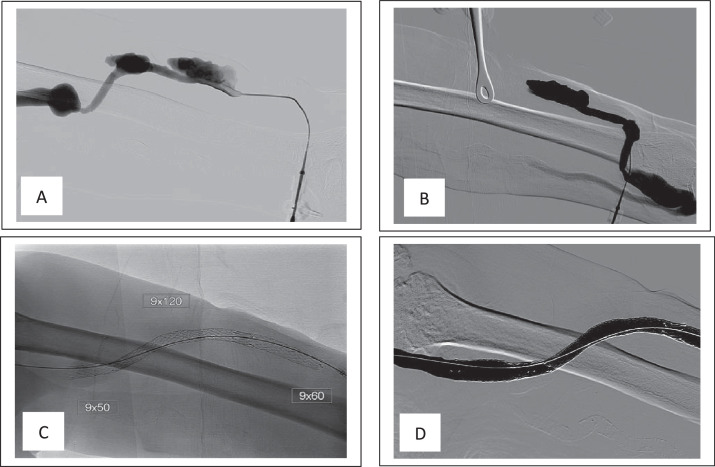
(A) graft angiogram showing 2 aneurysmal formations. (B) angiogram showing the arterial anastmosis and connection between cephalic vein and interpositioning graft. (C) single shot X-ray showing 3 overlapping stents. (D) poststenting angiogram revealing the exclusion of the aneurysmal formations.
